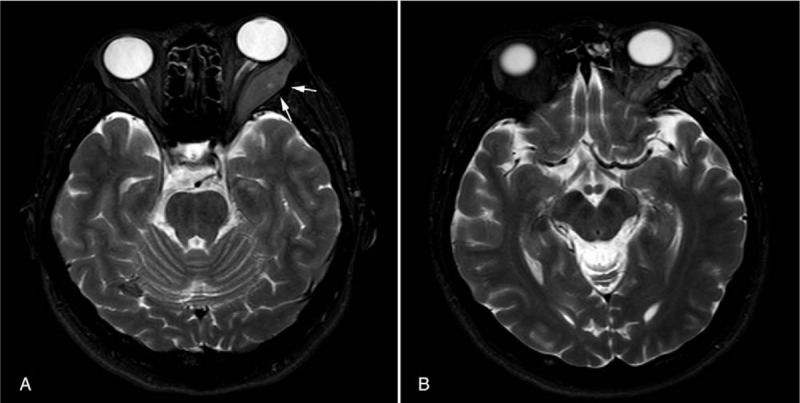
MRI revealed a mass of left lateral oculus. (A) before surgery (the mass is indicated by arrows, 3.6 × 1.3 cm). (B) after surgery. MRI = magnetic resonance imaging.
